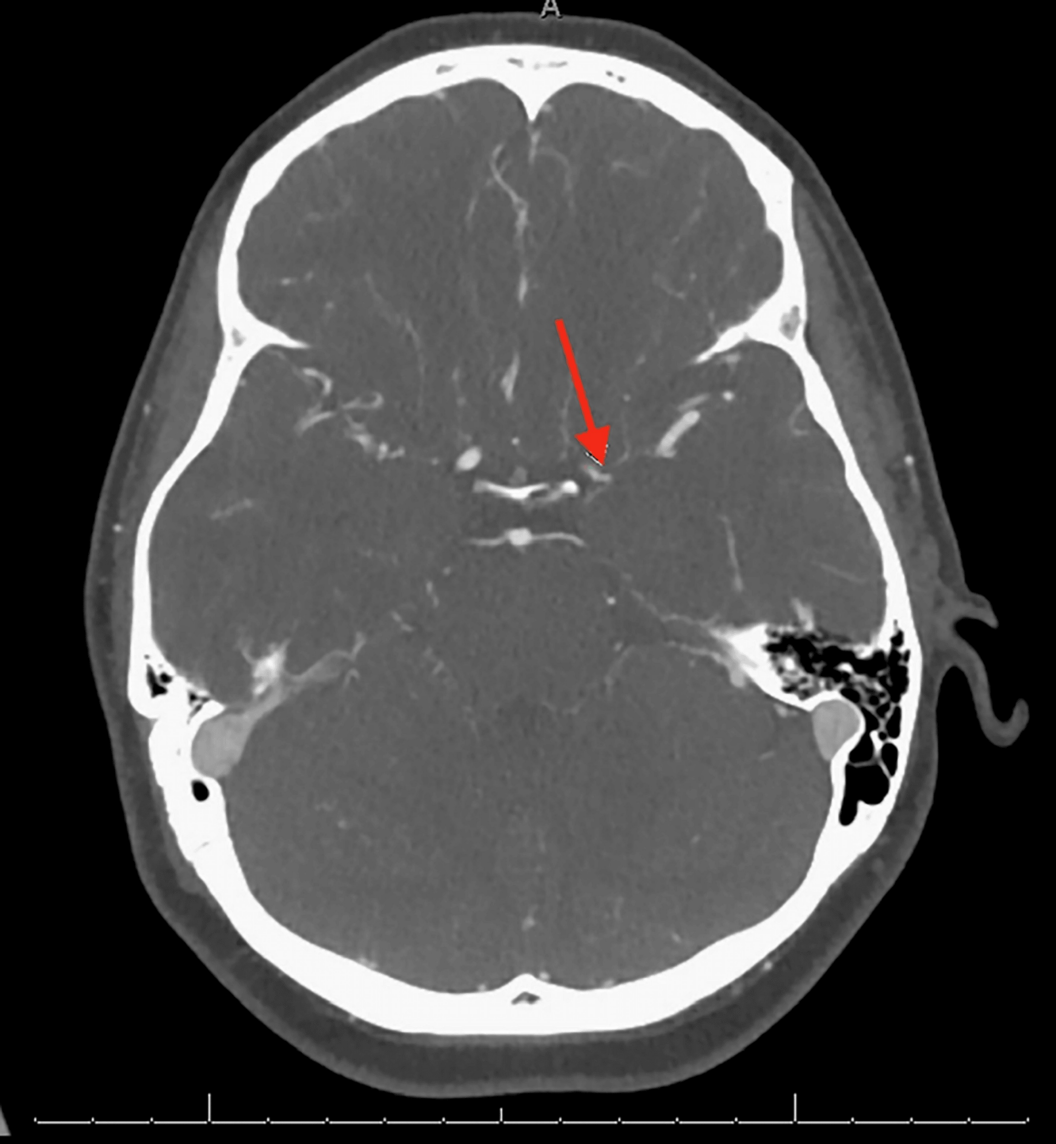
Computed tomography angiography of the head in a patient presenting with sudden-onset thunderclap headache and expressive aphasia The arrow indicates persistent focal high-grade stenosis of the distal left supraclinoid internal carotid artery (ICA) of at least 50% according to the Warfarin–Aspirin Symptomatic Intracranial Disease (WASID) study criteria.
